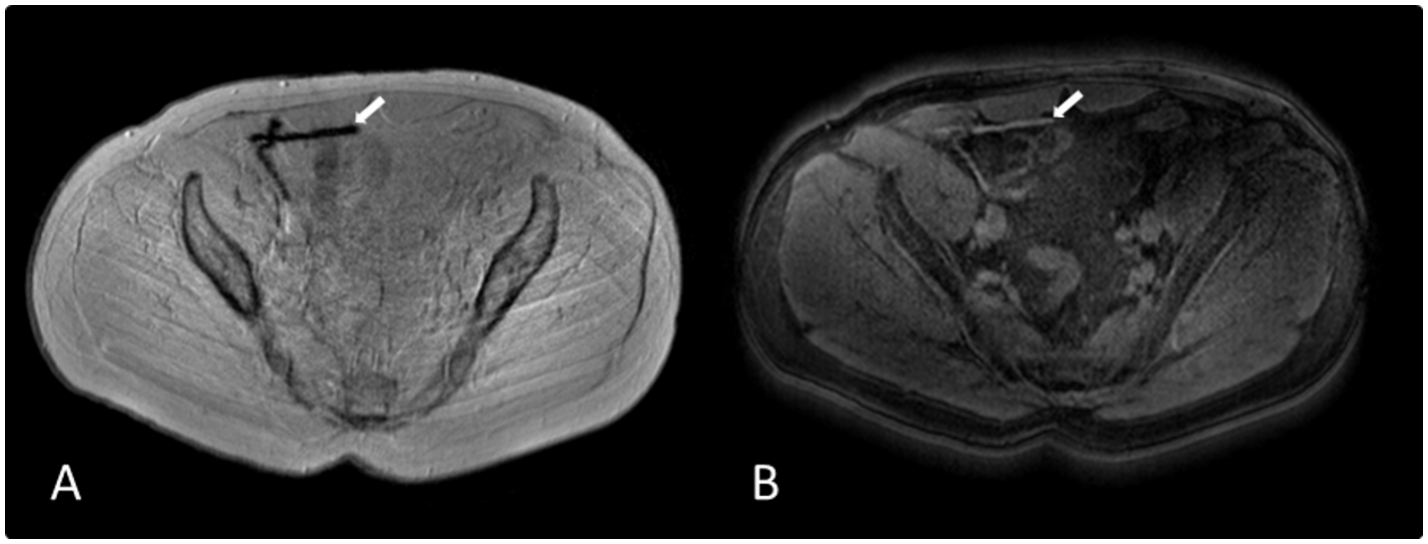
Transversely orientated MR images of a patient’s groin after laparoscopic hernia repair using a mesh implant (arrows). A: On GRE, the mesh-induced signal voids (arrow) clearly contrast to the surrounding hyperintense anatomy resulting in precise delineation. B: On PCSI, the susceptibility differences induced by the iron-loaded mesh implant (arrow) exhibit as hyperintense signals.
